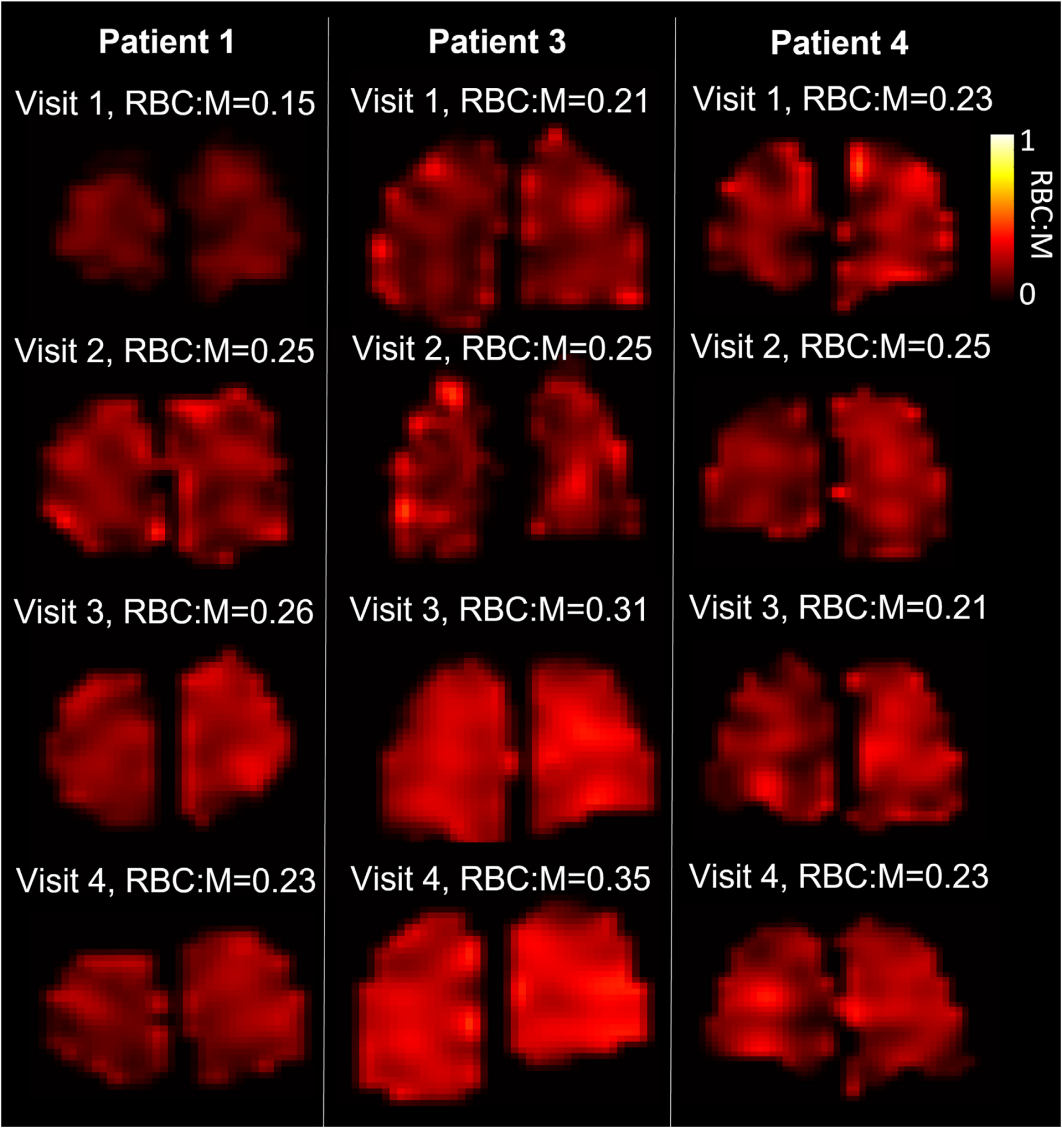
Lung RBC:M maps in three patients with four MRI visits at 6, 12, 25, and 51 weeks following hospital admission. Mean RBC:M at each visit is shown. M = membrane; RBC:M = RBC to membrane fraction.
